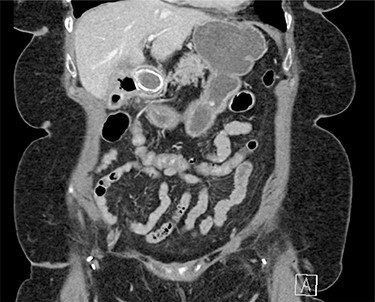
Abdominal CT scan (coronal plane) with the large impacted gallstone causing gastric outlet obstruction proximally with no small bowel dilation distally.
